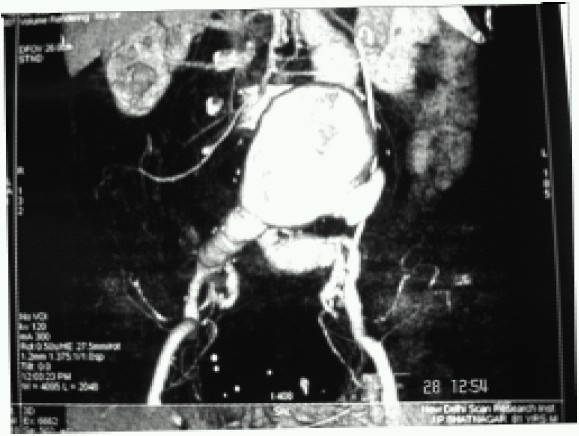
Spiral computed tomography showing an infrarenal aortic aneurysm with a contained leak (represented by arrow)
